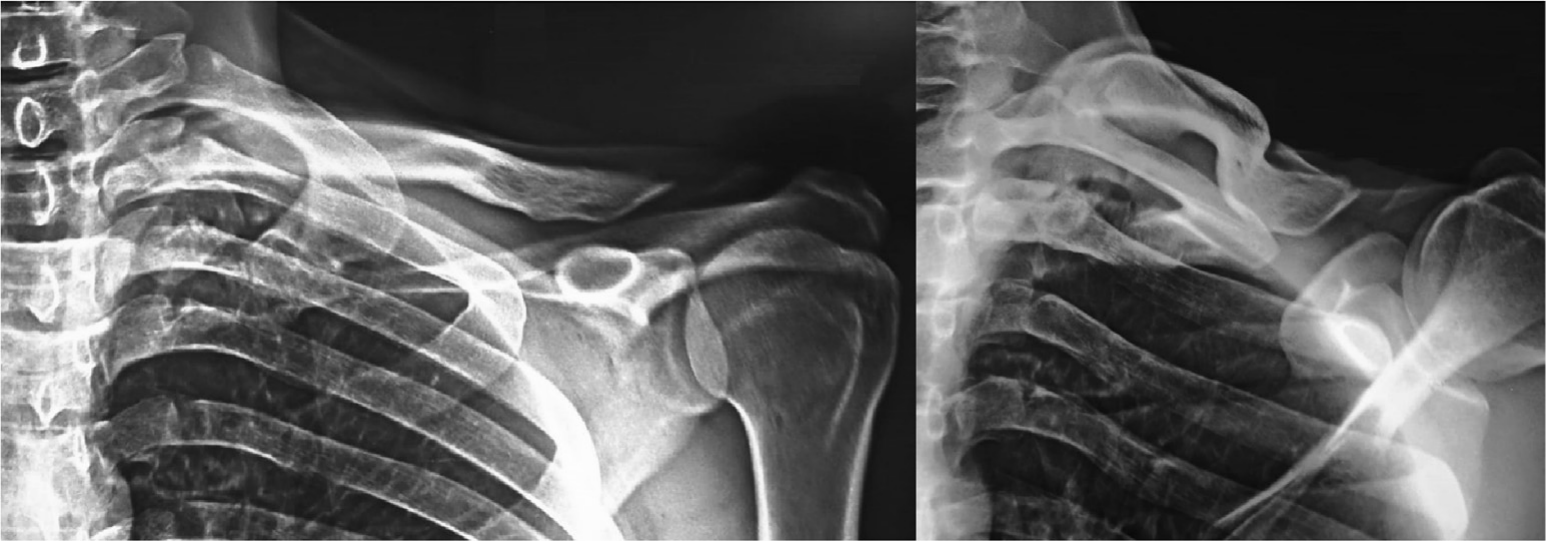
Preoperative radiographs of the shoulder girdle showing bipolar dislocation of the clavicle.
